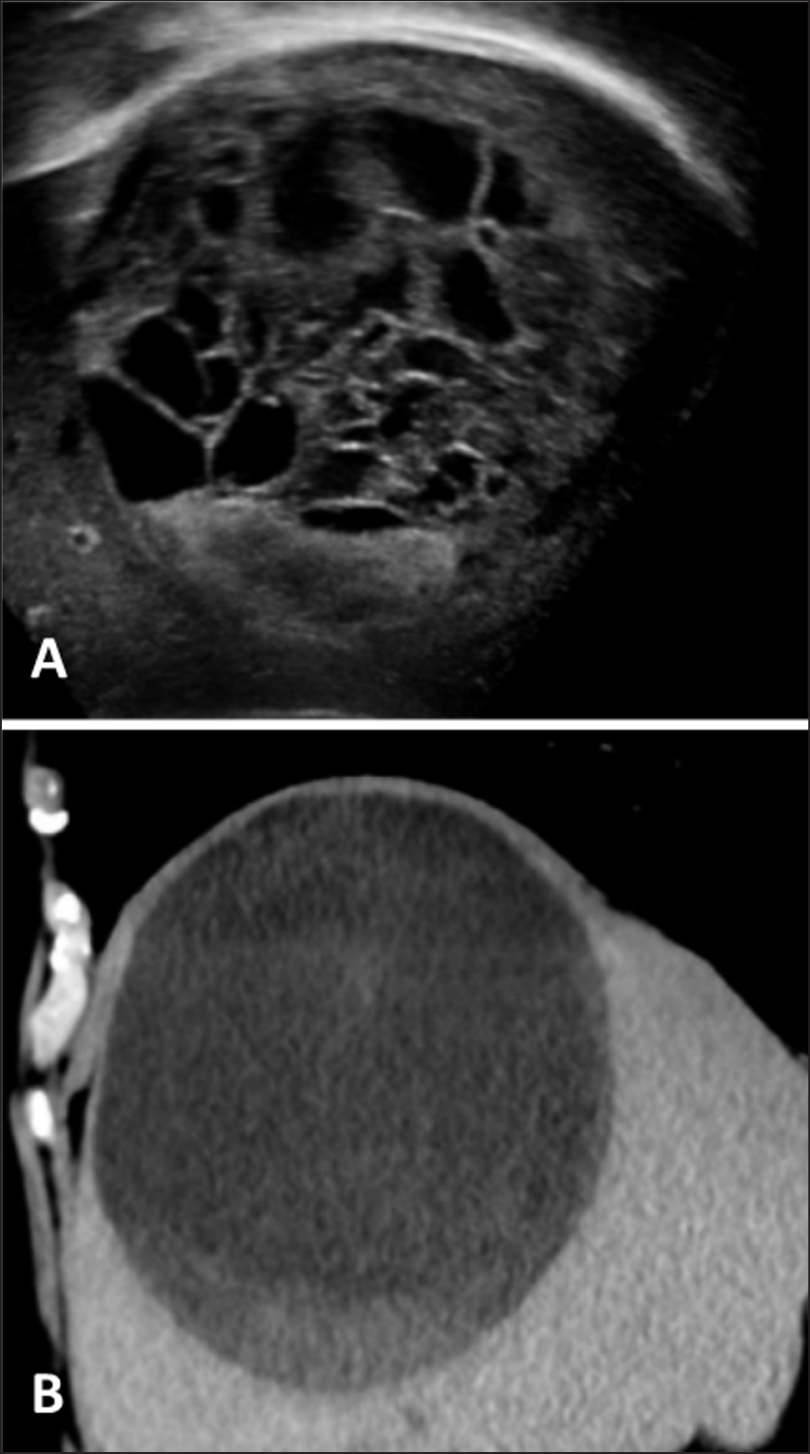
An MRI performed two months after initial assessment demonstrated a significant volume decrease of this hemorrhagic cyst. Changes of the cyst content signal confirmed its hemorrhagic nature: hyperintense on T1-weighted sequences without and with fat saturation, with a fluid-fluid level (Figure 3a). On T2-weighted images, the major portion in the superior part showed an intermediate signal, whereas the lower part is hypointense, suggesting a blood clot. The cyst is surrounded by a rim of hypointensity on T2- and T2*-weighted sequences corresponding to haemosiderin (Figure 3b). After the administration of Gadolinium, there is no enhancement.
